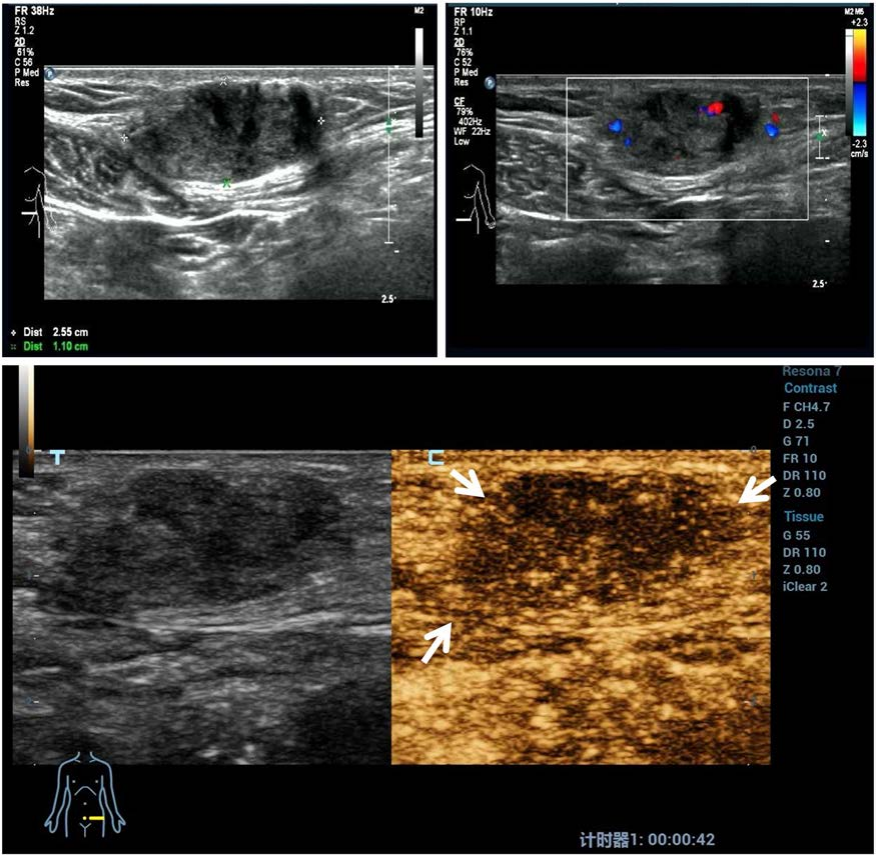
A representative case before ultrasound-guided microwave ablation. A 41-year-old patient had a hard mass on a transverse cesarean incision with cyclic abdominal wall pain. Ultrasound examination of the AWE mass revealed a heterogeneous mass with ill-defined, blurred outer margins. Color Doppler ultrasound images show scarce flow within the mass. The contrast-enhanced ultrasound imaging of the AWE nodules shows slight perfusion compared with the surrounding tissue. AWE, abdominal wall endometriosis.
